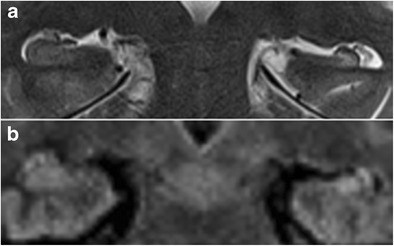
Left-sided mesial temporal sclerosis. 3 T coronal T2-weighted (a) and FLAIR images (b) in a 43-year-old patient with medically refractory epilepsy show volume loss, increased signal intensity and blurring of the internal structure of the left hippocampus, compatible with mesial temporal sclerosis
